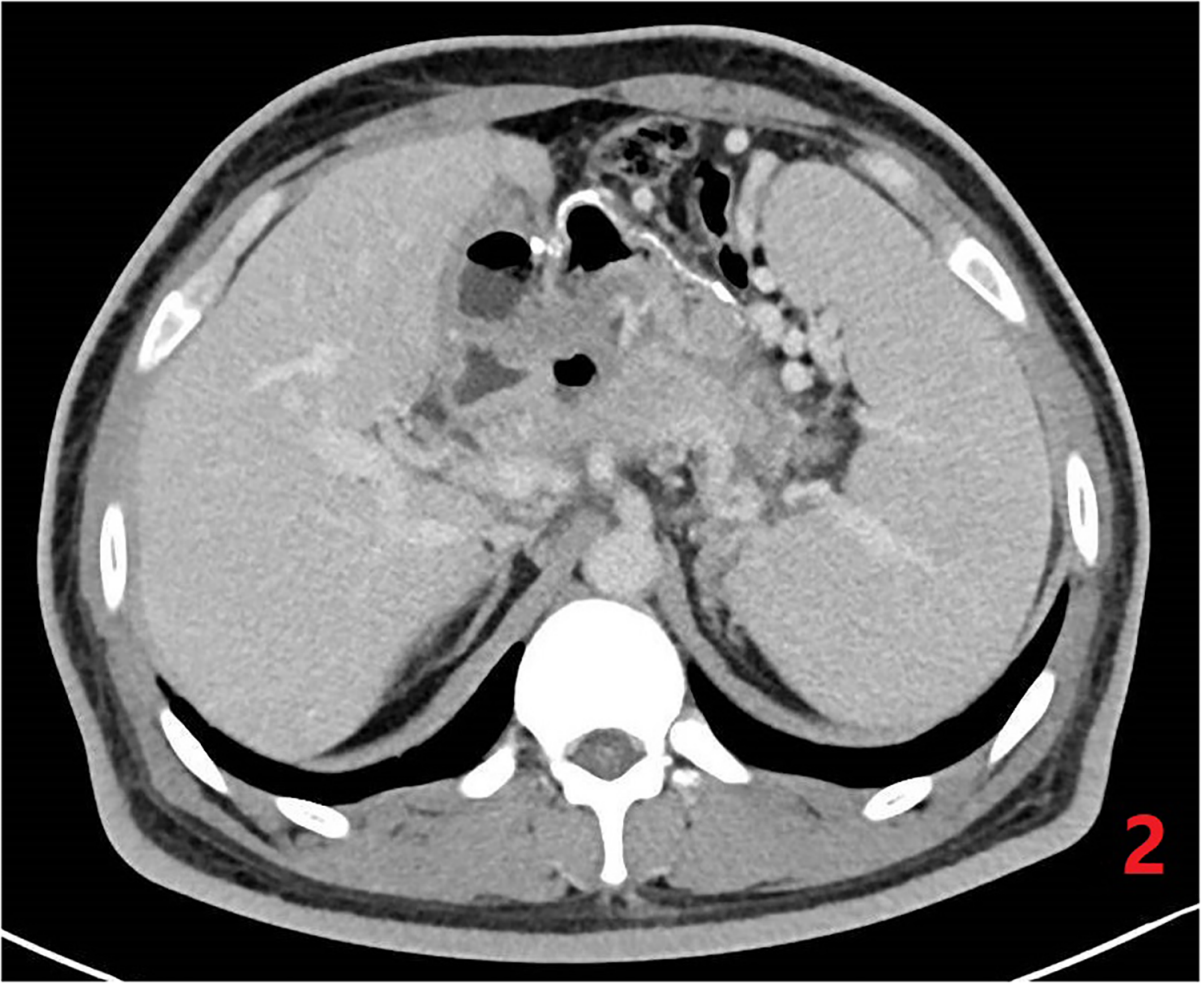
Postoperative CT of abdomen. Resolution of pseudocyst, but with regional portal hypertension.
